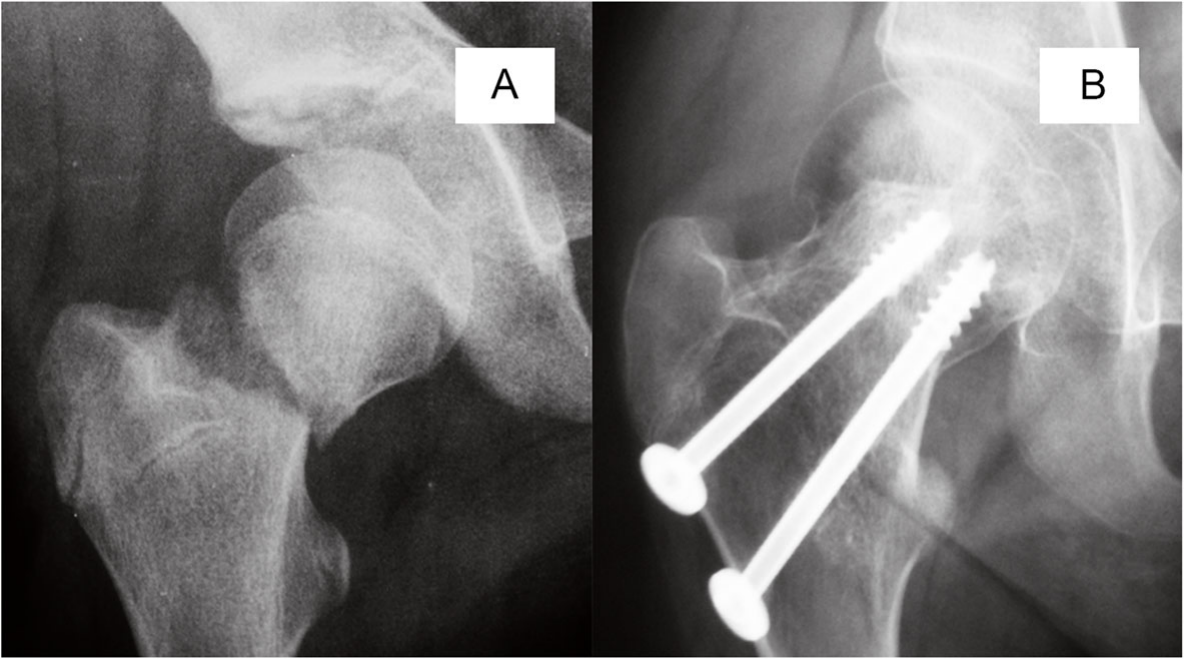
The anteroposterior radiographs of Ratliff type II avascular necrosis after pediatric femoral neck fracture. Femoral neck fracture occurred at age of 10 years (a) and avascular necrosis were diagnosed 5 months later (b), and JIC type C1, presenting non-collapsed femoral head
